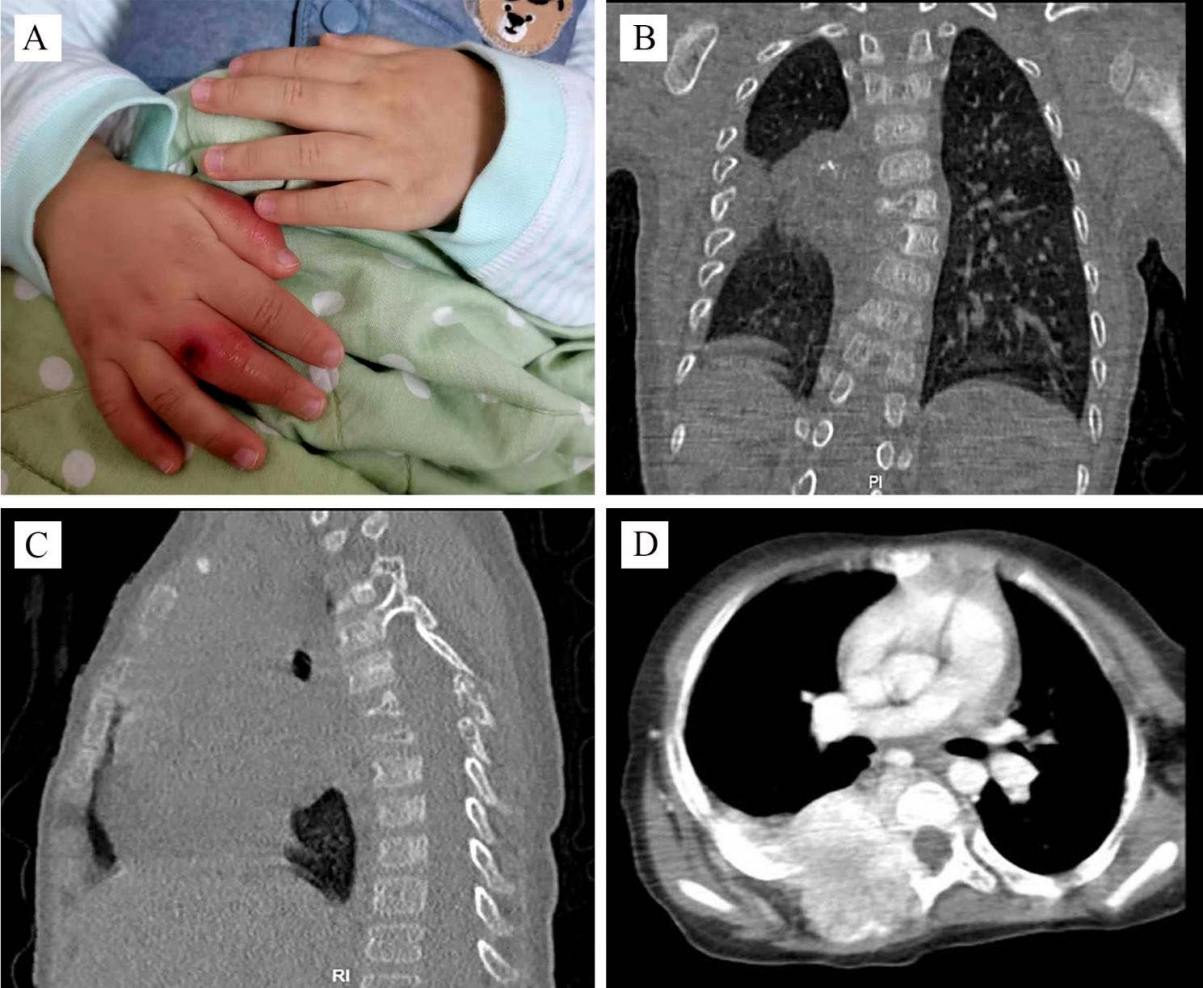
(A) Noted the swelling and frostbite-like rash of the right hand. The chest CT (B and C) showed an irregular mass on the right side of the spine (T3-6 vertebral level), presenting with bone destruction of vertebral, attachments, and multiple ribs. The enhanced CT (D) scan shows an enhanced soft-tissue mass extending to the posterior thoracic musculature and the spinal canal
